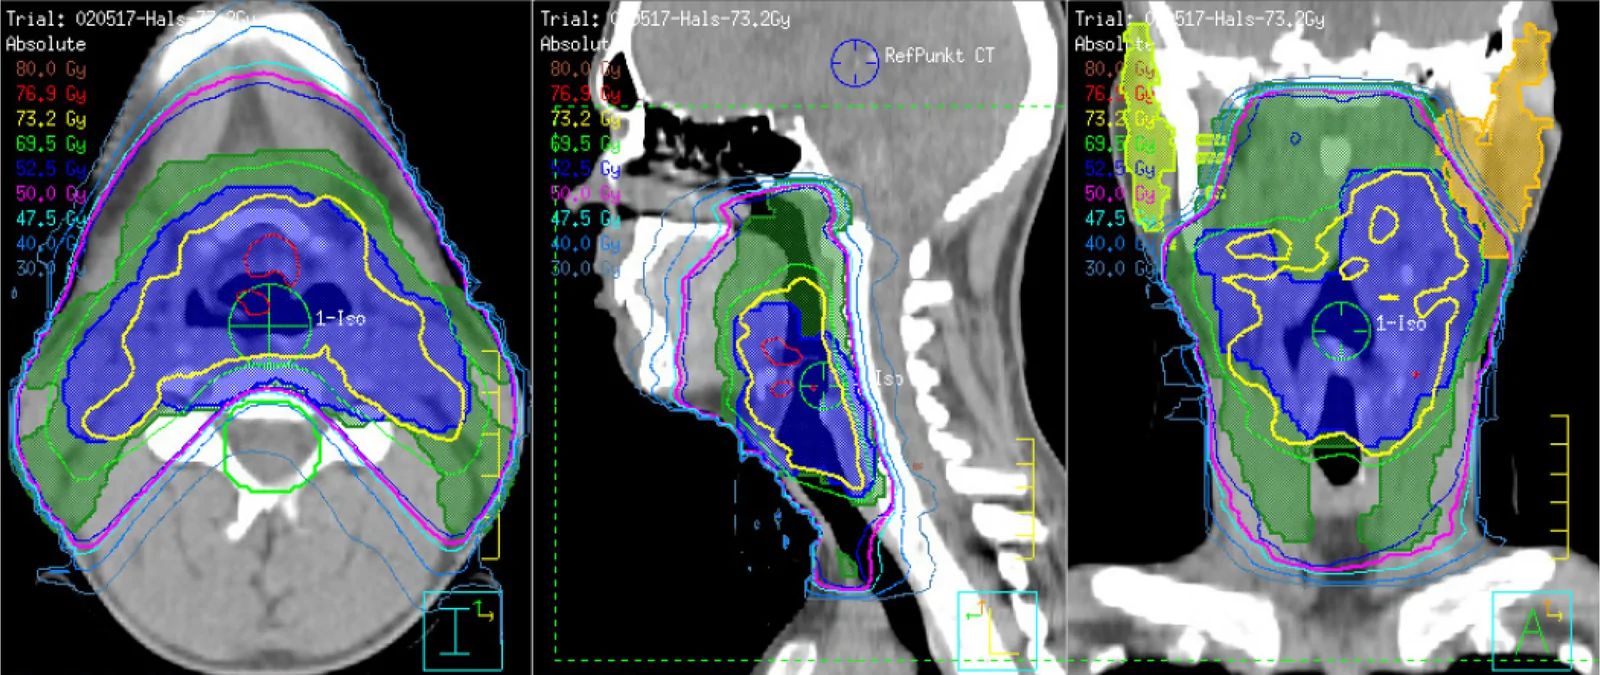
Intensity-modulated radiotherapy (IMRT) treatment plan: PET/CT-based target volumes with cumulative 73.2 Gy to initial gross disease (primary and nodes) and 54 Gy to elective regions
